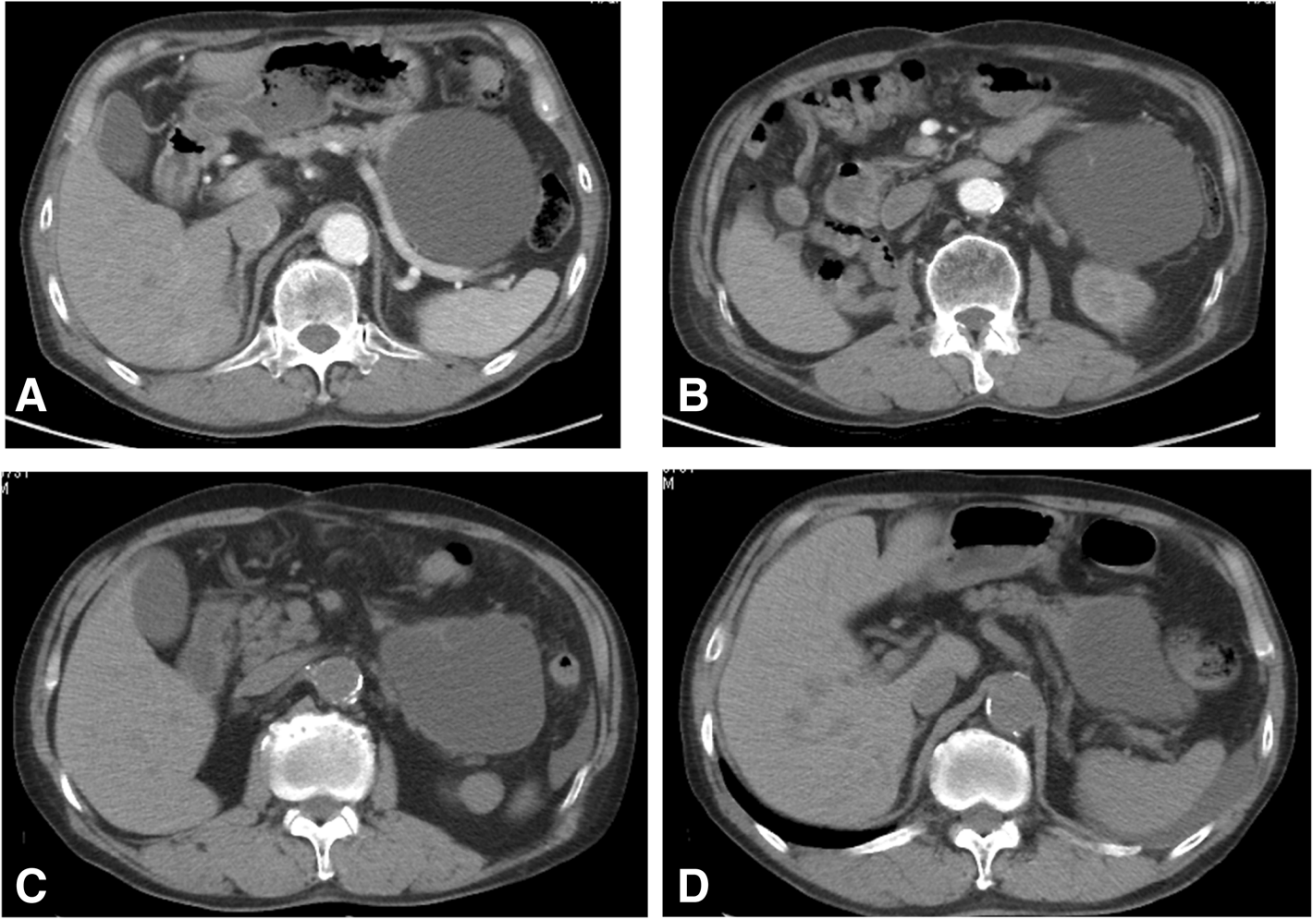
Prior computed tomography findings. In 2014, the pancreatic cyst size was 103 mm (a, b). In 2015, the pancreatic cyst ruptured, and the patient was treated conservatively (c, d)
